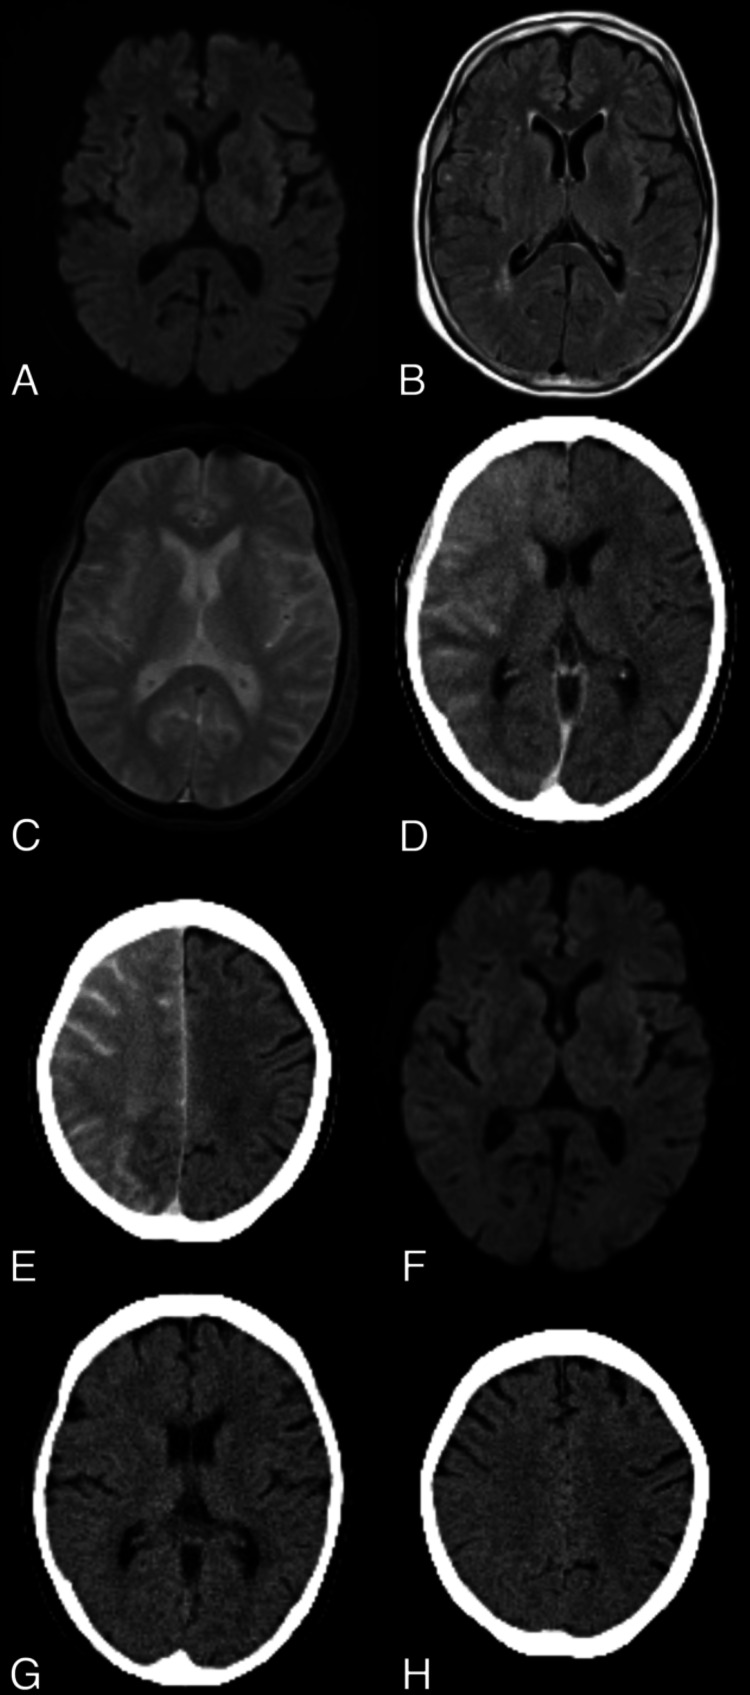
Postoperative MRI and CT findings DWI (A), fluid-attenuated inversion recovery (B), and T2*-weighted imaging (C) revealed no acute ischemic or hemorrhagic changes. Noncontrast CT, performed within one hour after coil embolization, showed cortical and subcortical contrast enhancement in the right cerebral hemisphere (D and E). DWI on postoperative day one (F) showed no ischemic changes. The contrast enhancement in the right cerebral hemisphere on CT completely resolved by postoperative day four (G and H). DWI, diffusion-weighted imaging
